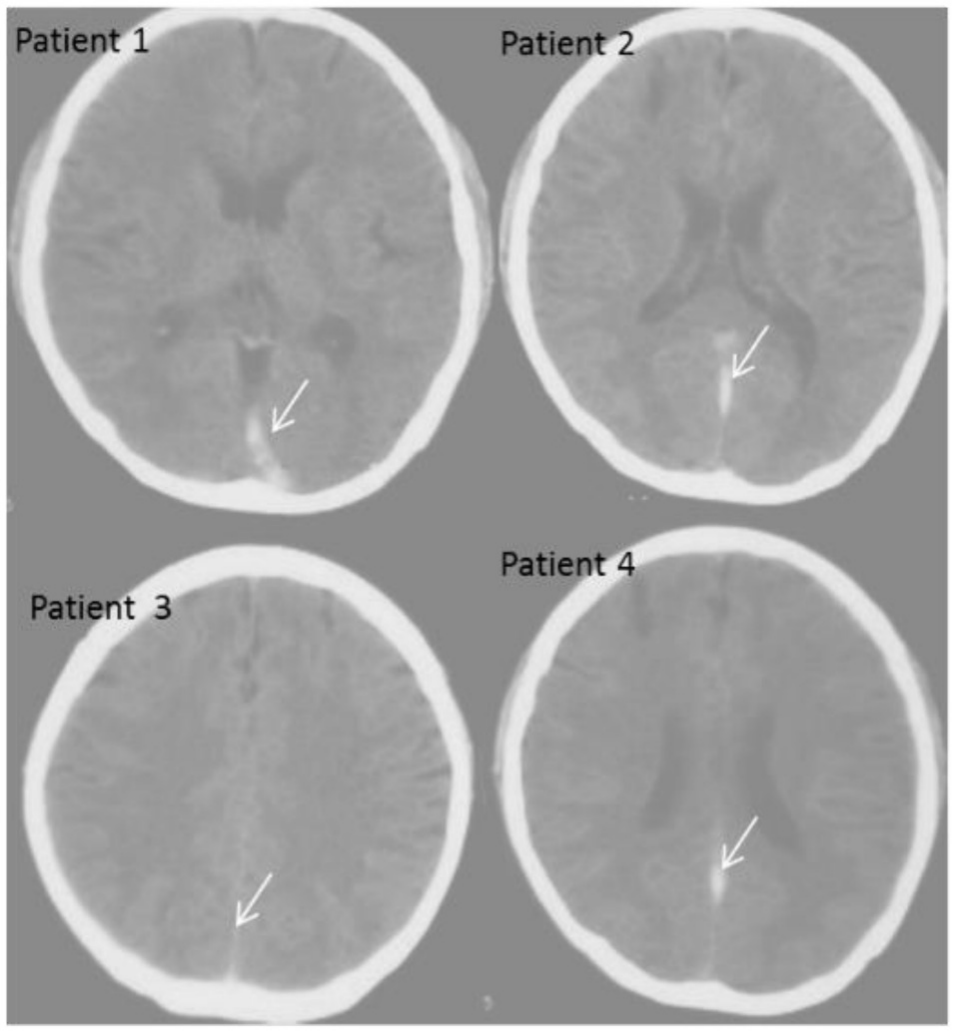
CT diagnosis of the patients with CVT. Patient 1, a male patient aged 36; Patient 2, a female patient aged 40; Patient 3, a male patient aged 34; Patient 4, a female patient age 35.
